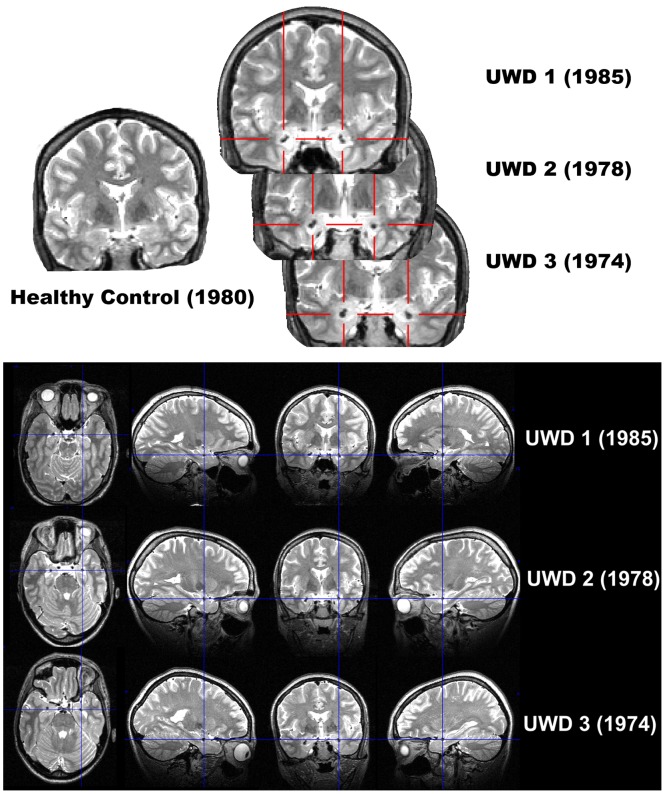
Structural MRI scans showing bilateral amygdala calcification in UWD. Figure 2a: Coronal view T2-weighted MR-images of the three UWD subjects and one control subject with year of birth. Crosshairs indicate calcified brain damage. Figure 2b: T2-weighted MR-images in all three planes of the three UWD subjects. Crosshairs indicate the location of calcified brain damage bilaterally.
